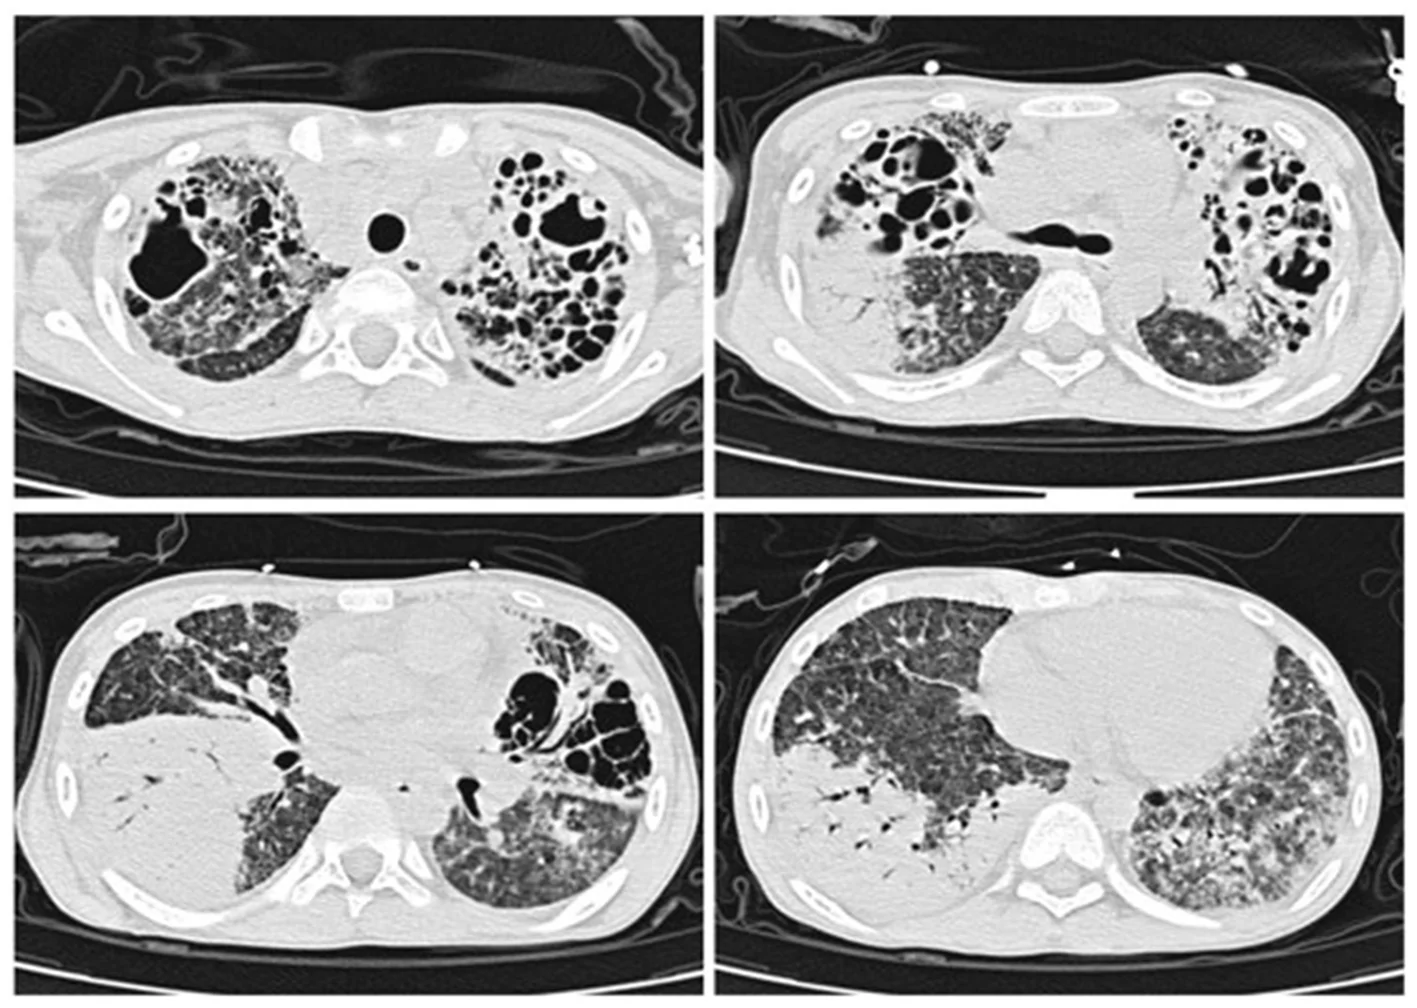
Chest computed tomography (CT) of the patient. Transverse CT images revealed bilateral pulmonary infiltrates, right lower lobe consolidation, cystic lesions in both upper lobes, and mediastinal lymphadenopathy.
